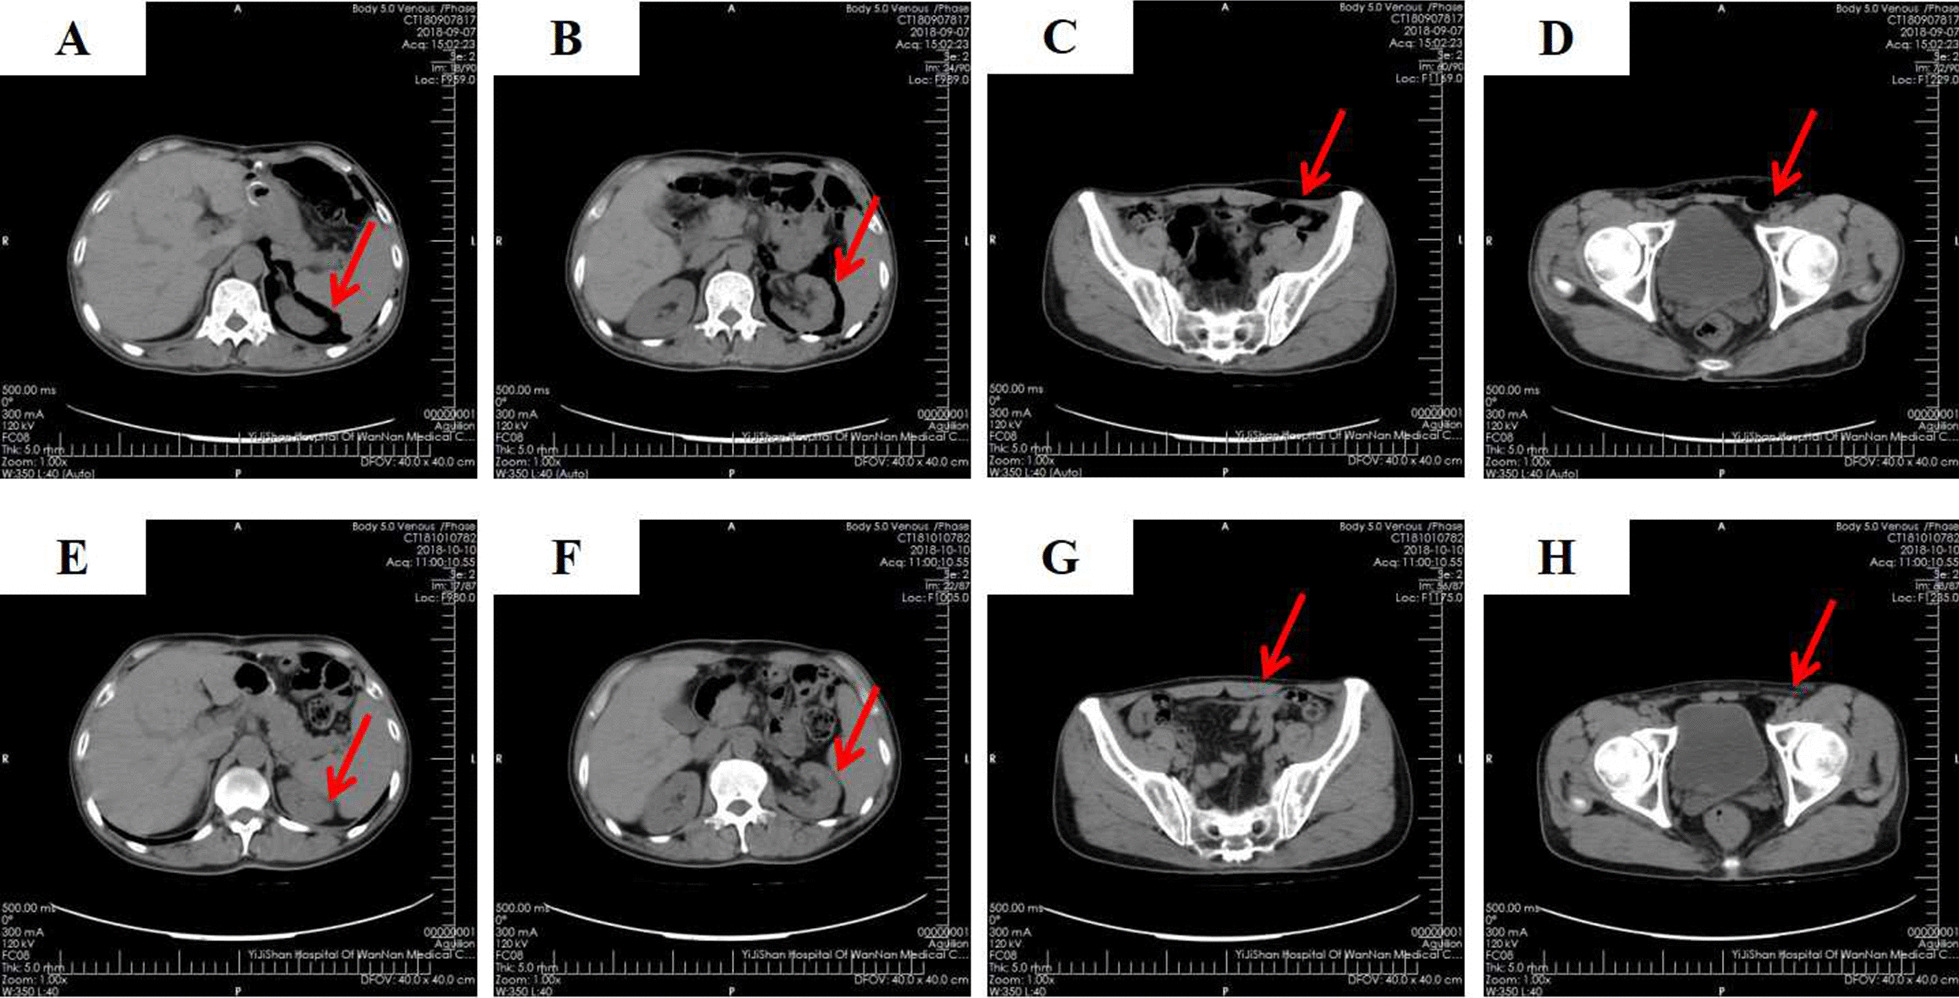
Perirenal pneumatosis A, B and subcutaneous emphysema of the abdominal wall C, D were obvious on CT 3 days after OLIF surgery (red arrow). One month later, the perirenal pneumatosis E, F and subcutaneous emphysema of the abdominal wall G, H had almost completely disappeared
